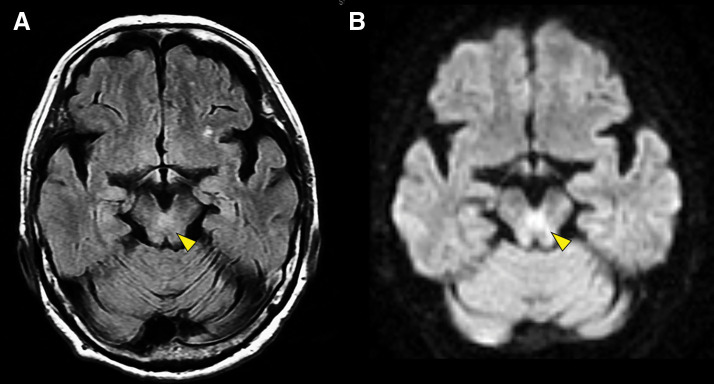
Brain MRI. Hyperintensity (yellow arrowheads) is demonstrated around the periaqueductal region of the midbrain on (A) fluid-attenuated inversion recovery and (B) diffusion-weighted imaging.
